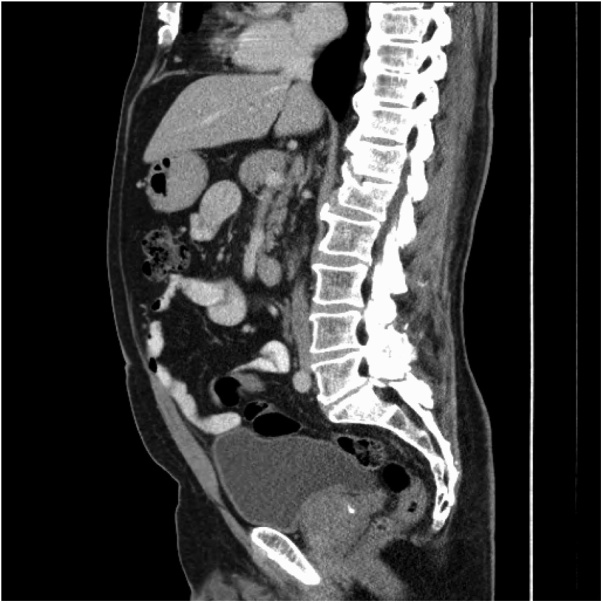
Sagittal view of CT AP showing 4.5 cm lobulated mass in the anterior abdominal wall.
